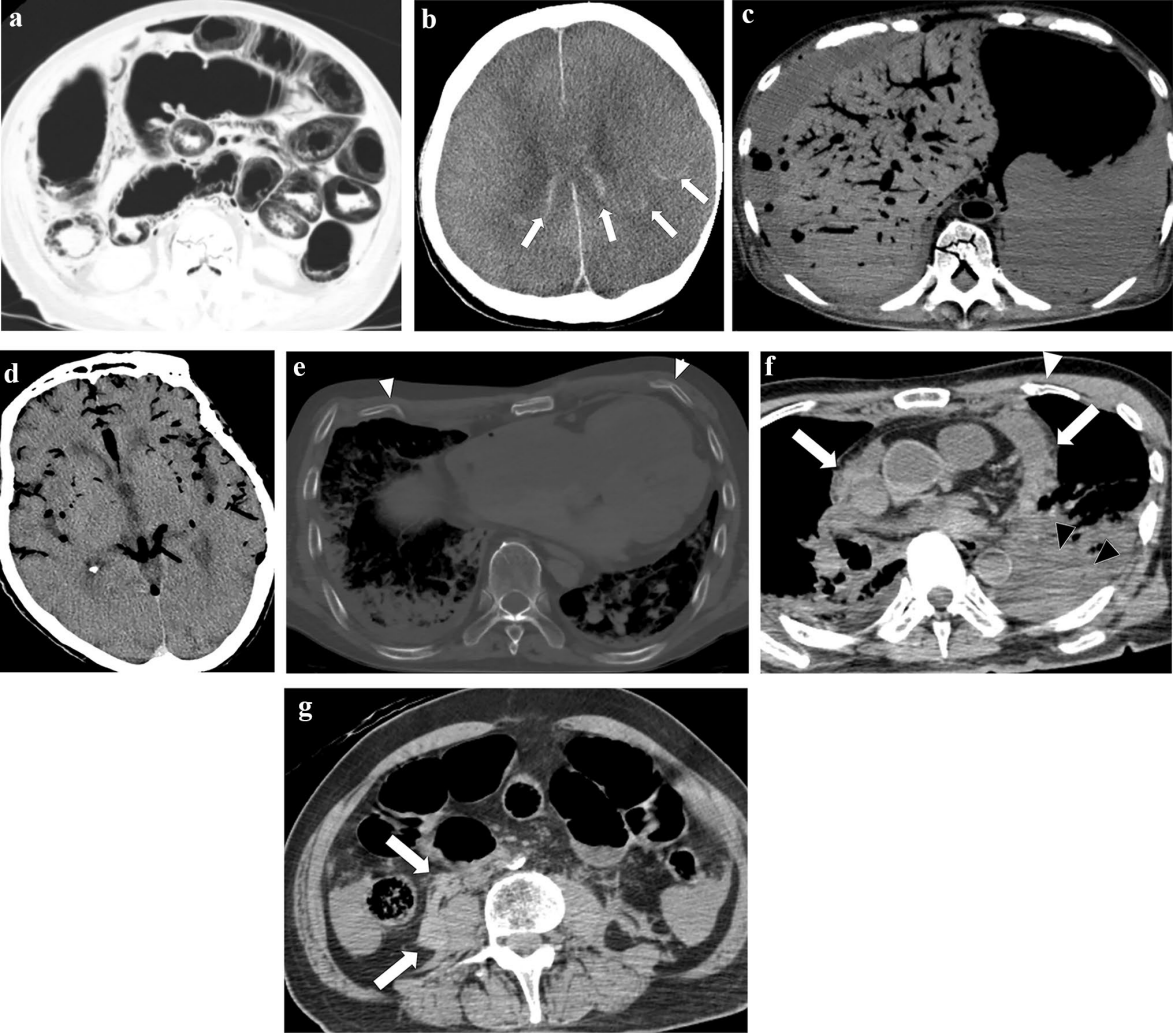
Representative CT images reflecting cardiopulmonary resuscitation. a extensive dilation of the gastrointestinal tract, gas in the intestinal wall and mesenteric vasculature. b the dilated medullary veins (arrows) near the lateral ventricles. c intraorganic and intravascular (broadly observed in the liver, abdominal aorta, vertebral body, and spinal canal) gas (scanned 10 h after death and also observed abdominal free air due to possible autolysis (unidentified bowel perforation by autopsy)). d gas in the arteries and veins in the brain (same case as Fig. 3c). e rib fractures in the anterior thoracic regions (arrowheads). f hemopericardium (arrows) due to cardiac rupture and sequel left hemothorax (black arrowheads) (also observed left rib fractures in the anterior thoracic regions (white arrowhead)). g right retroperitoneal hemorrhage (arrows) due to unknown cause confirmed by autopsy (same case as Fig. 3f)
